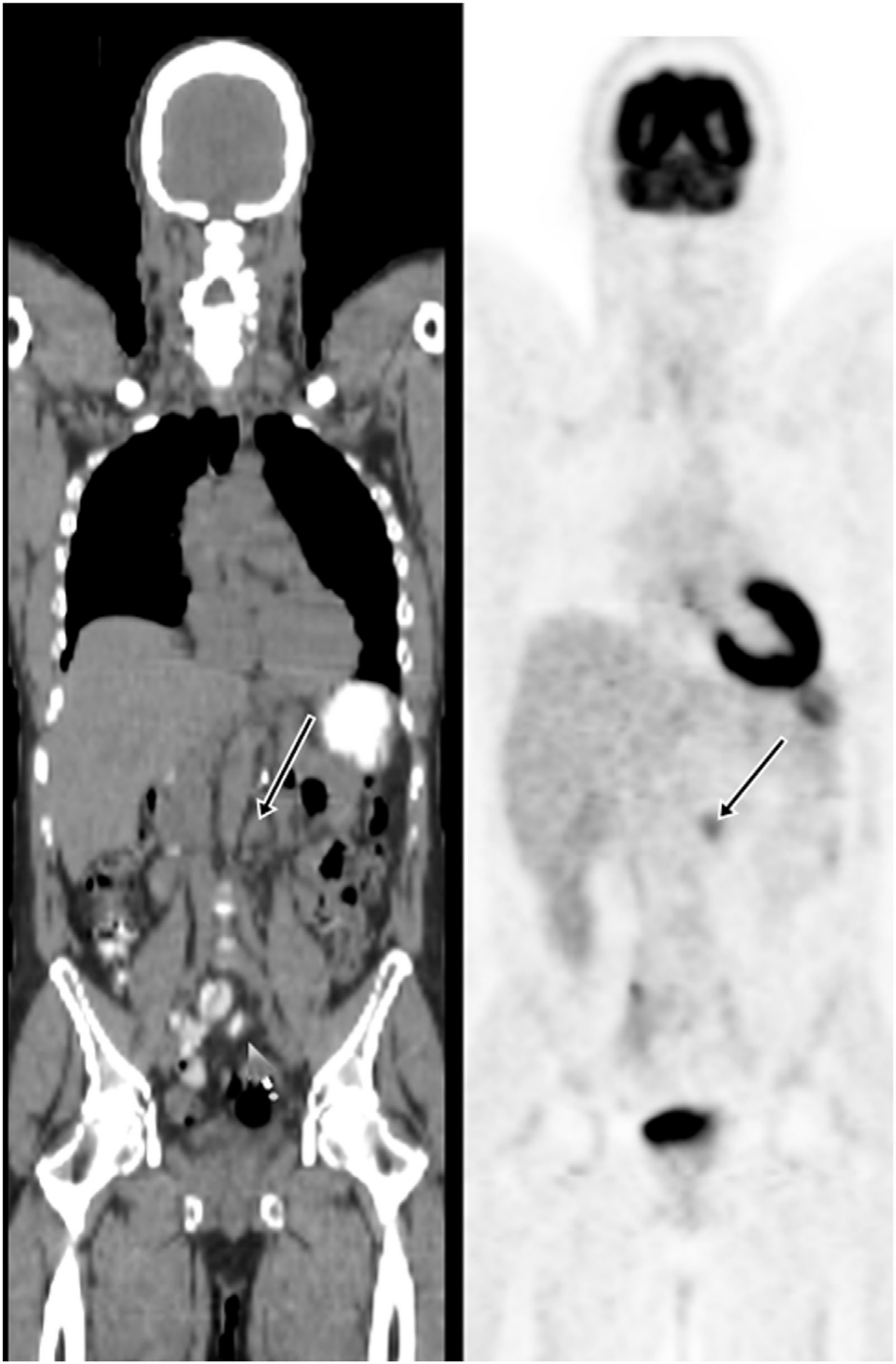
Demonstration of metastatic lymph node on FDG PET/CT. A 57-year-old man had the left nephrectomy for RCC 5 years ago. A restaging FDG PET/CT showed a 1.5 cm left para-aortic node with moderate uptake. Subsequent node dissection confirmed metastasis.
